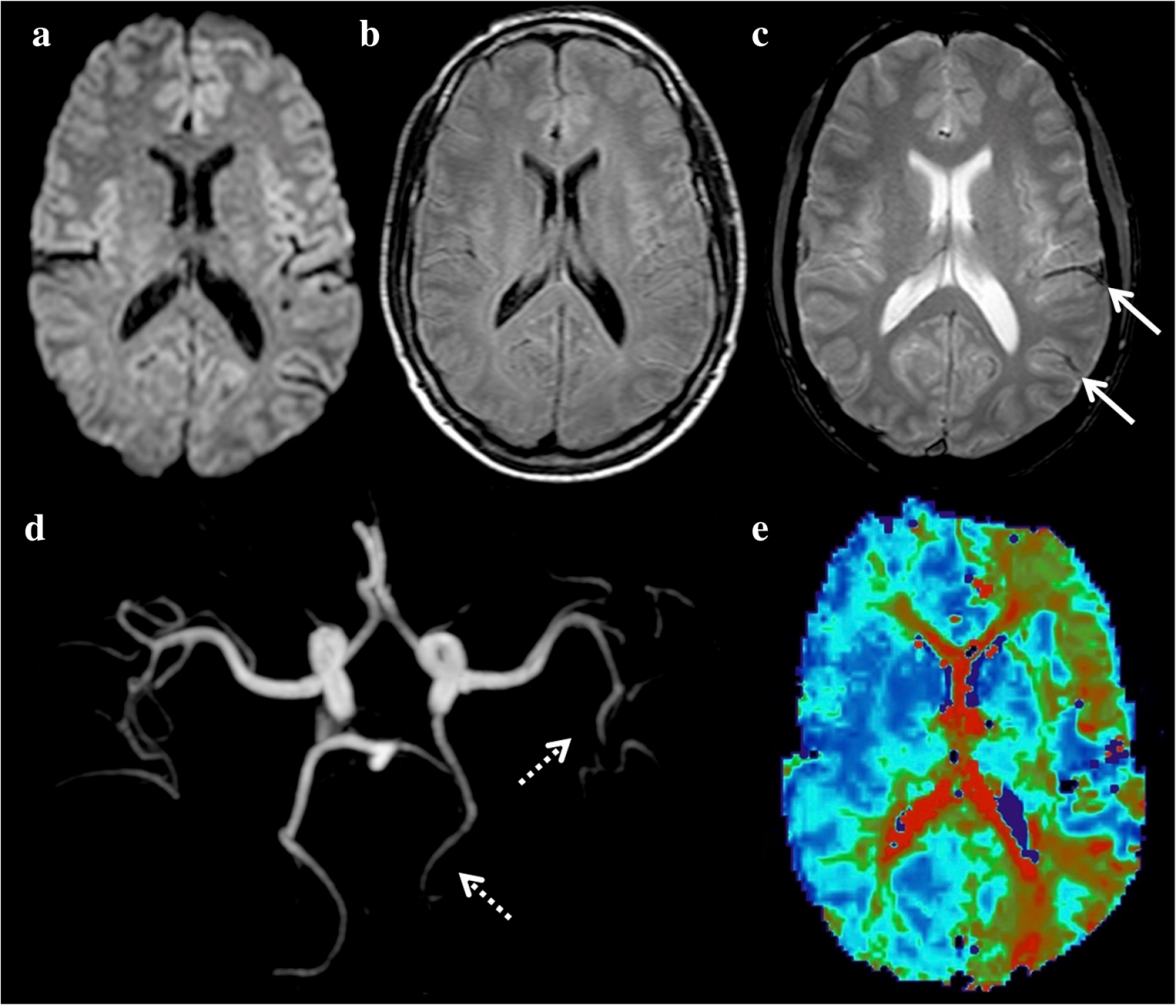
A 38-year-old man with migraine aura presenting with motor, sensory and language deficits. DWI shows no hyperintensity (a). FLAIR is unremarkable (b). Hypointense signals of the venous on T2* (c, arrows). Regional and distal spasm of the arteries on TOF-MRA (d, dotted arrows) in relation with the hypoperfused areas with delayed TTP (e)
